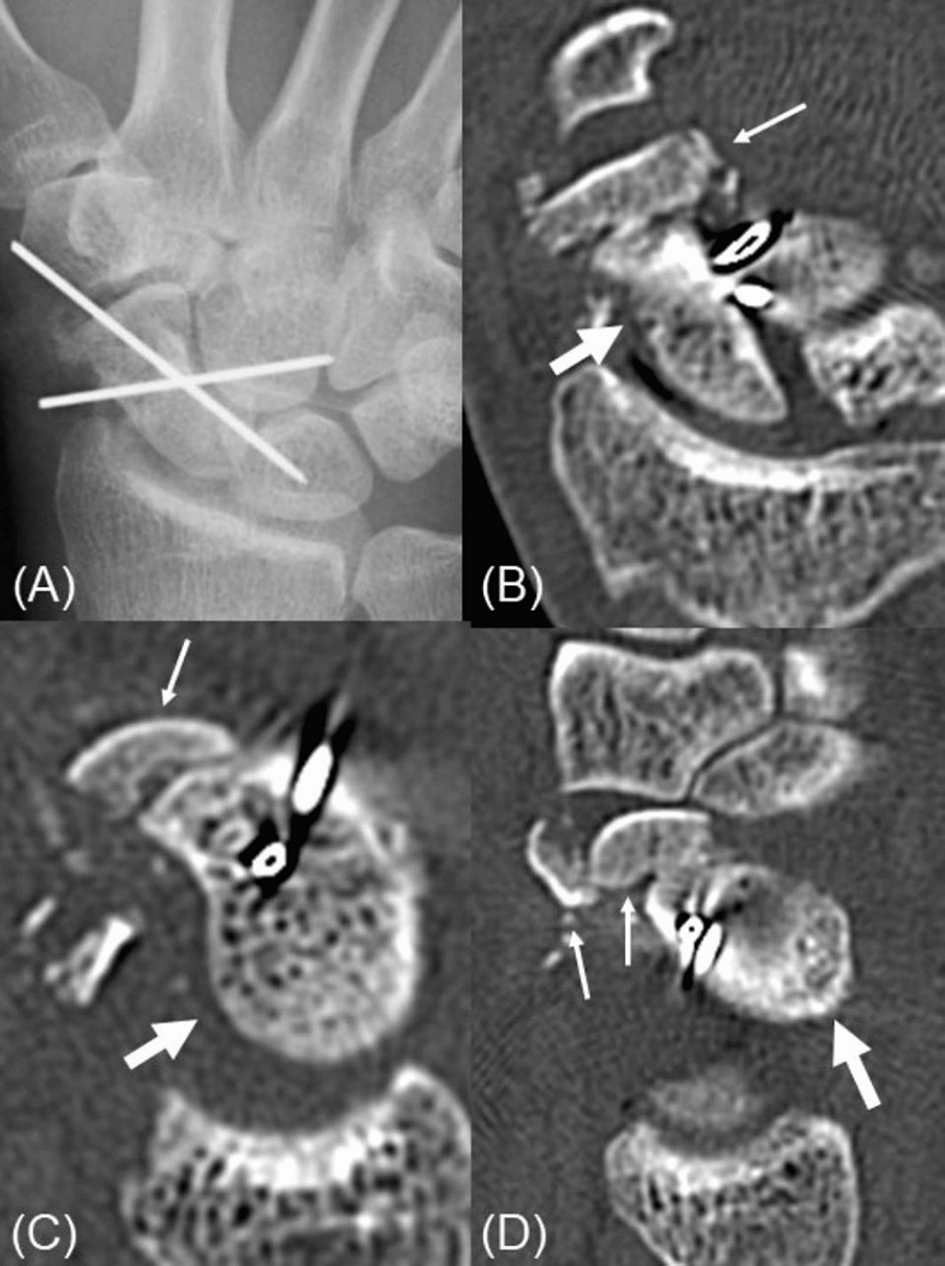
Radiographs and CT scans after initial treatment. Radiographs and CT scans after initial treatment display reduction and temporary fixation. The thick arrows show the proximal fragment of the scaphoid. The thin arrows show the distal fragment of the scaphoid. (A) Antero-posterior film of the left wrist shows the reduction of dislocation of the proximal fragment of the scaphoid and temporary fixation with Kirschner wires. (B-D) Coronal and sagittal CT images show that the dislocation of the proximal fragment of the scaphoid has been reduced, but the distal fragments are comminuted and thin.
